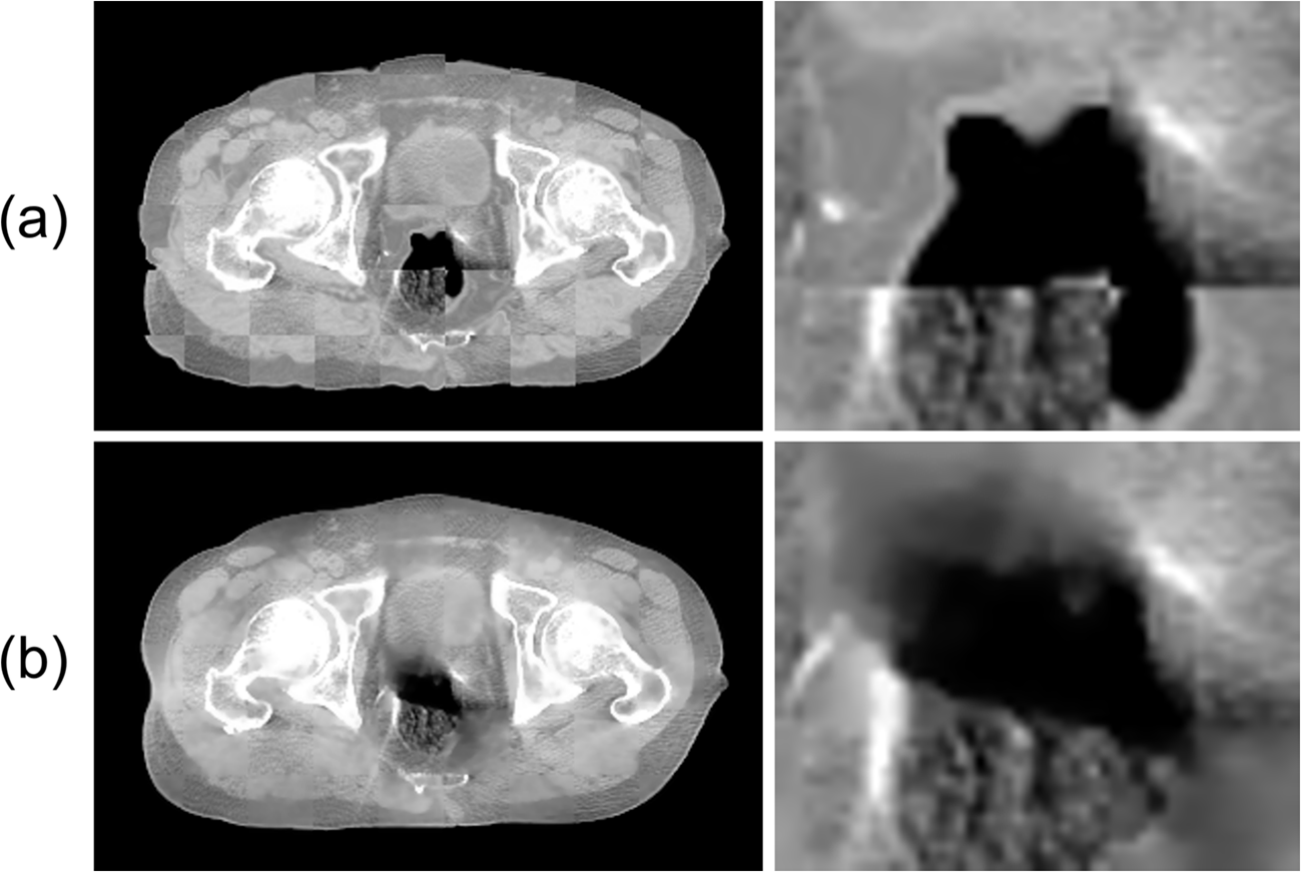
Checkerboard images. Checkerboard image of CBCT image and treatment planning CT image after B-spline deformable registration (a). Checkerboard image of CBCT image and SR(CBCT)_out_. Each right image is an enlarged rectal area (b) image. The CT number display window ranges from [− 840, 600] HU
